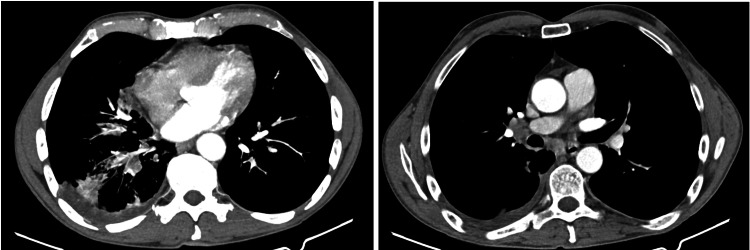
CT scan showing tree-in-bud pattern with consolidation (left) and hilar lymphadenopathy (right)
